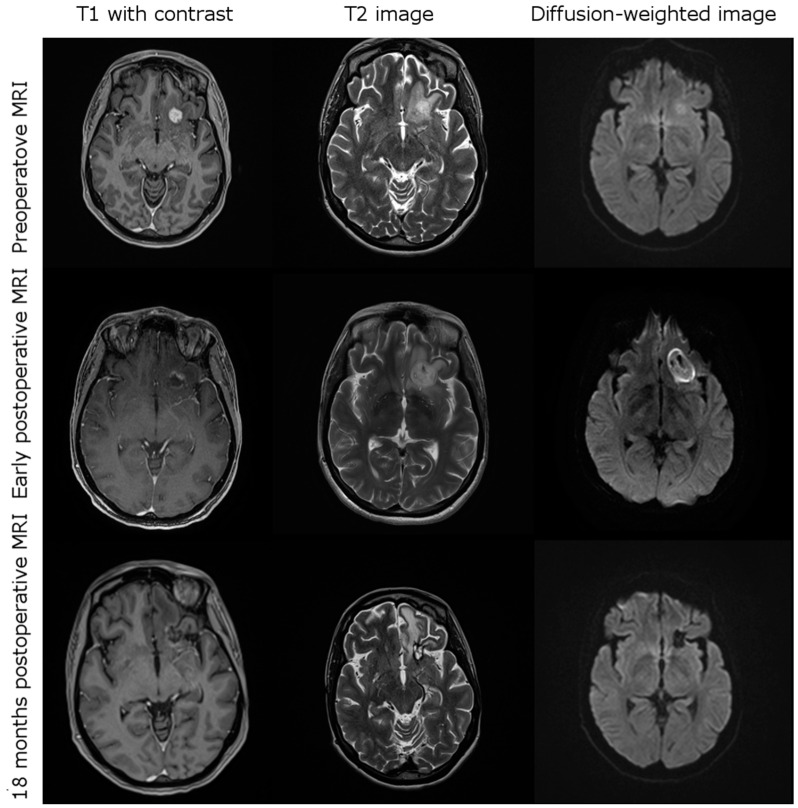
Exemplary case of a 41-year-old female patient suffering from a left frontal glioblastoma recurrence causing mild aphasia. Post-iPDT MRI showing characteristic changes such as disappearance of contrast enhancement, diffusion restriction within the treatment volume and mild perifocal edema. Peri-interventionally, there was transient worsening of aphasia, which then resolved completely in subsequent weeks.
